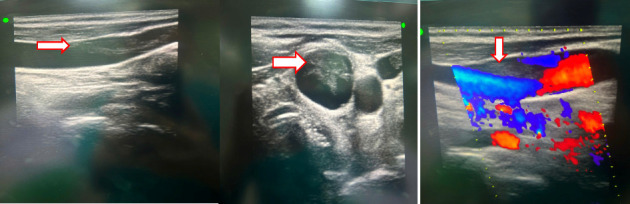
The ultrasound image shows left internal jugular vein thrombosis.
